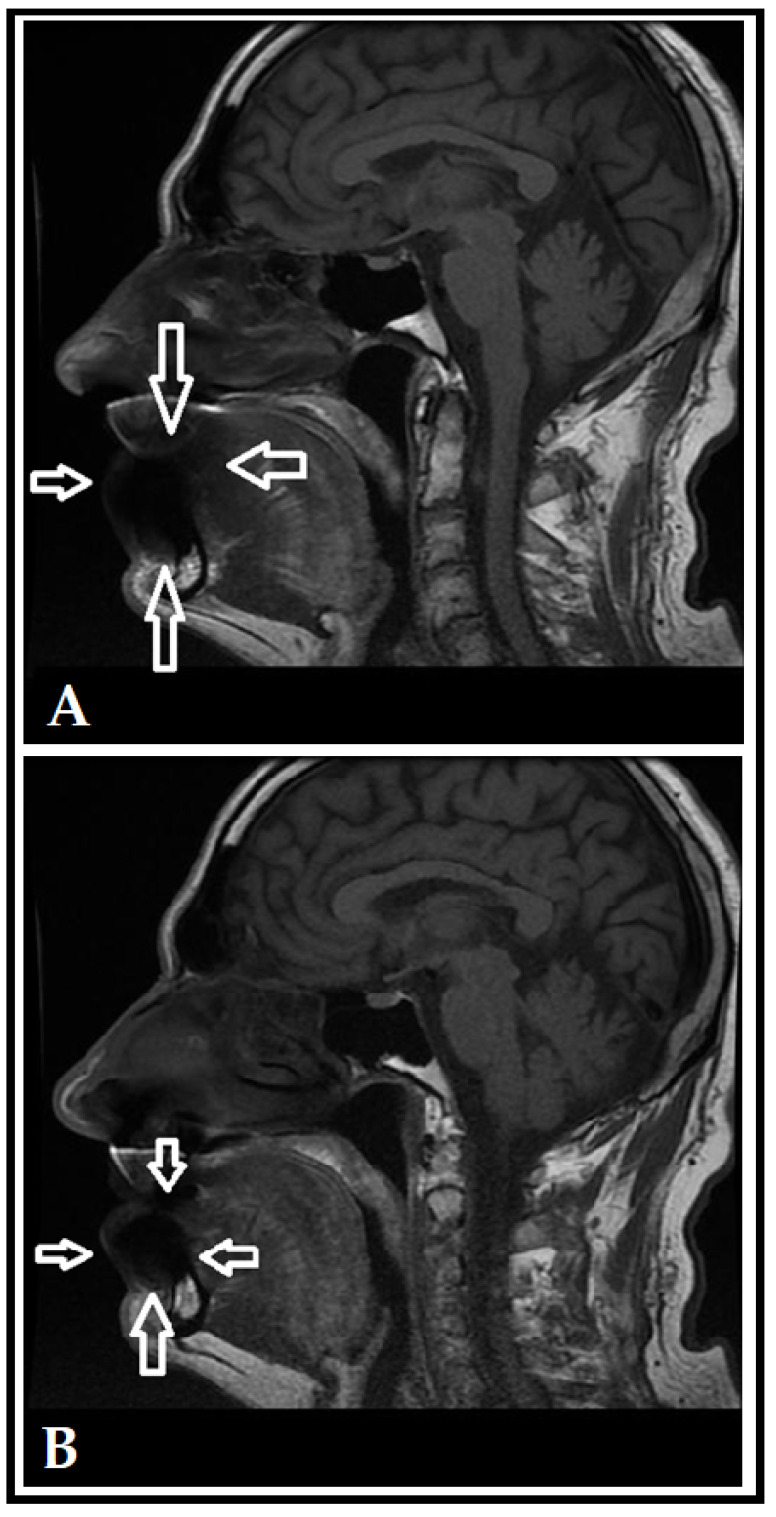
Unadjusted (A) and adjusted (B) MRI scans for Patient 1 (T1 sag). The shadow in the area of the lips (denoted by white arrows) indicates the imaging artefact caused by Cu accumulation in the lips, which is less pronounced after using adjusted directions and measurements.
